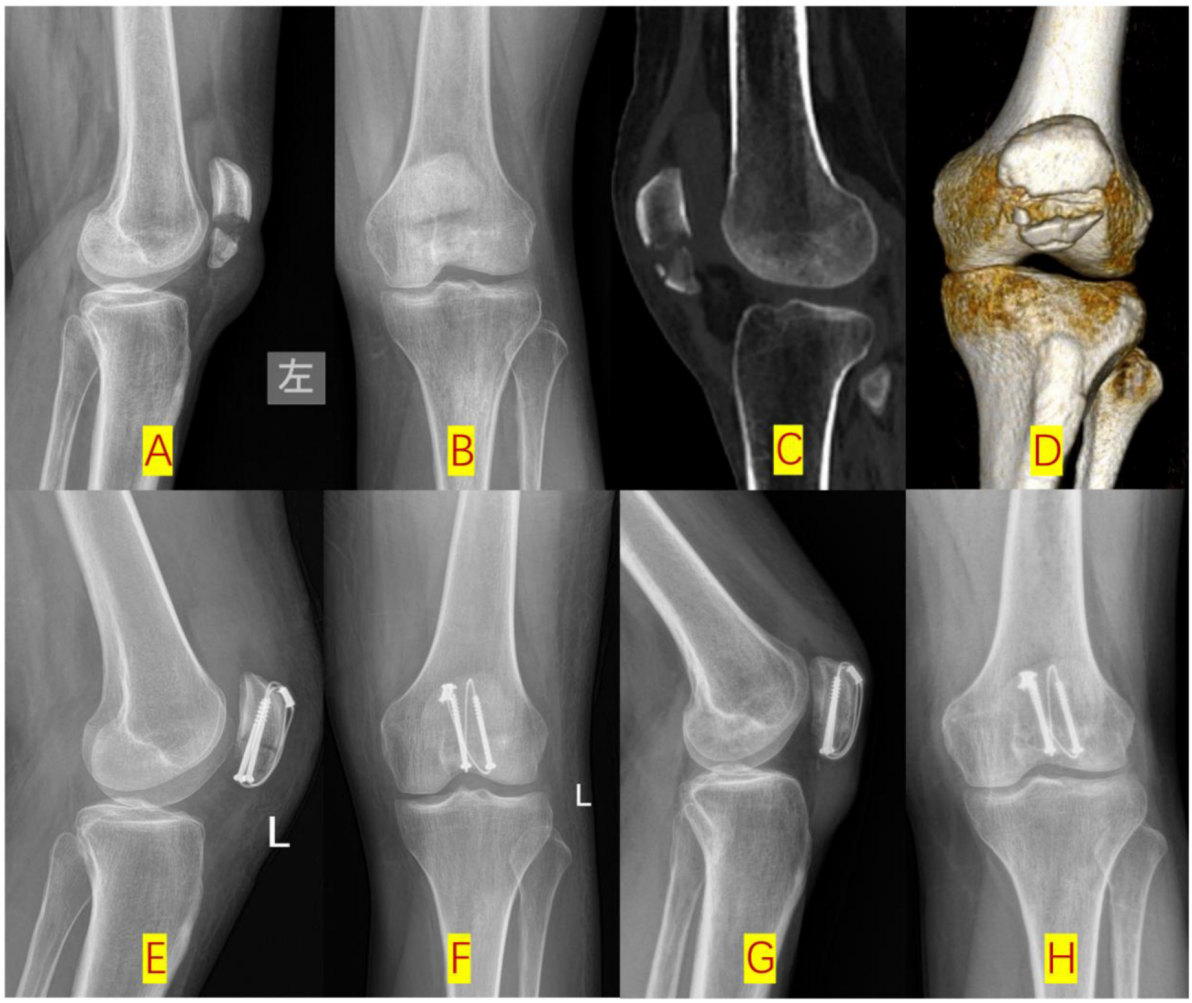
Type-C2 patellar fracture fixed by dual cannulated screw-cable system (DCSC) (A,B): Pre-op x-ray lateral radiographic and posteroanterior (PA) views; (C,D): Pre-op CT sagittal and 3D reconstructions; (E,F): post-op lateral and PA views; (G,H): 3-month post-op follow-up lateral and PA views.
